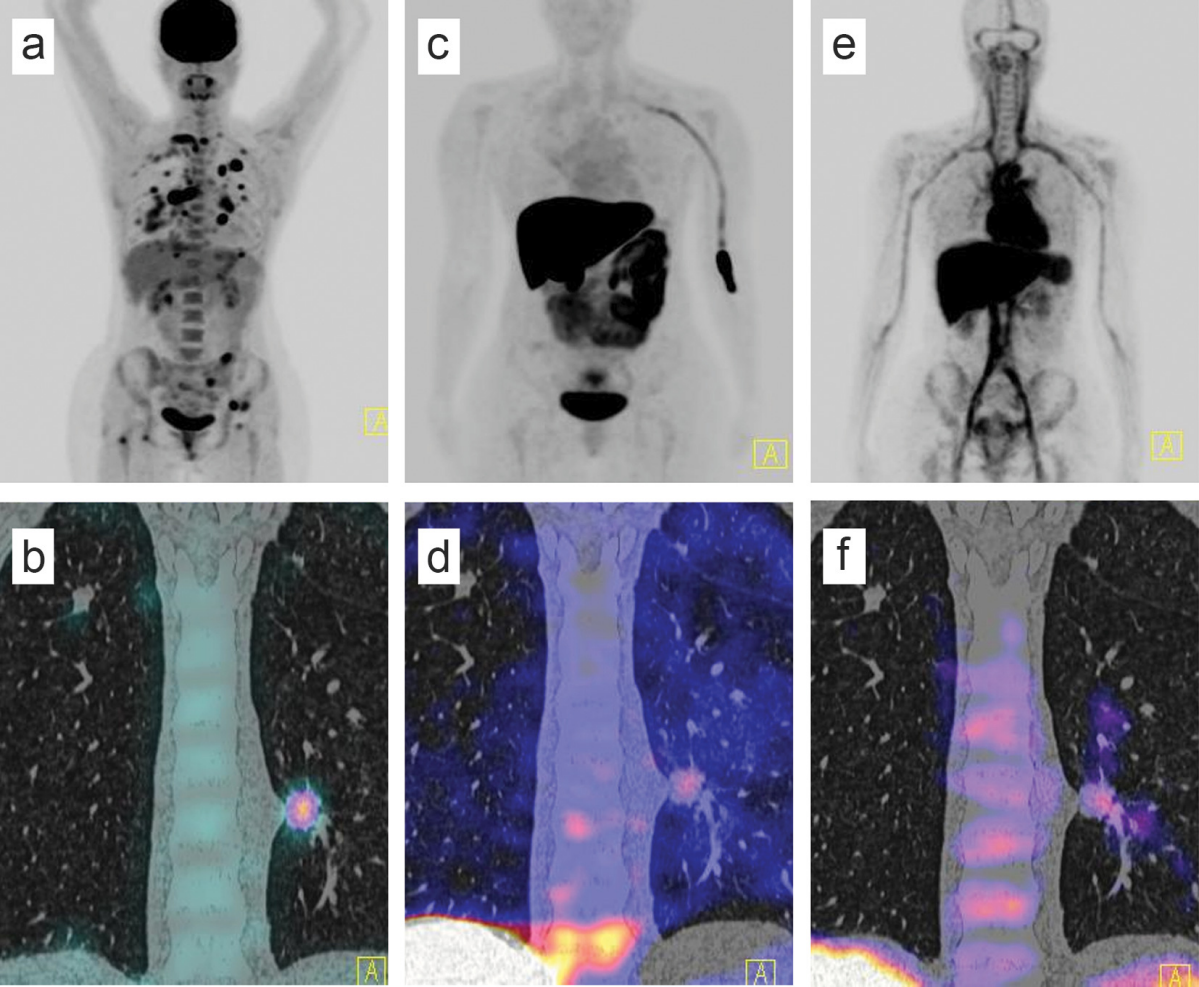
A 43-year-old woman with metastatic breast disease.^18^F-Fludeoxyglucose (FDG) PET/CT maximum intensity projection shows ^18^F-FDG-avid uptake in the lung, mediastinum and bone (a). Coronal PET/CT slice shows a 17 mm-pulmonary nodule localized at the posterior basal segment of the lower side of the left lobe with SUVmax of 10.7 (b). The patient was subject to another PET/CT study using ^18^F-fluoroestradiol (c). The maximum intensity projection shows focal uptake sites in the lung (d). Consistent with the metabolic response seen in panel (b), the same focus had an increased response by using ^68^Ga-DTPA anti-HER2 (SUVmax = 3.3) (e, f).
